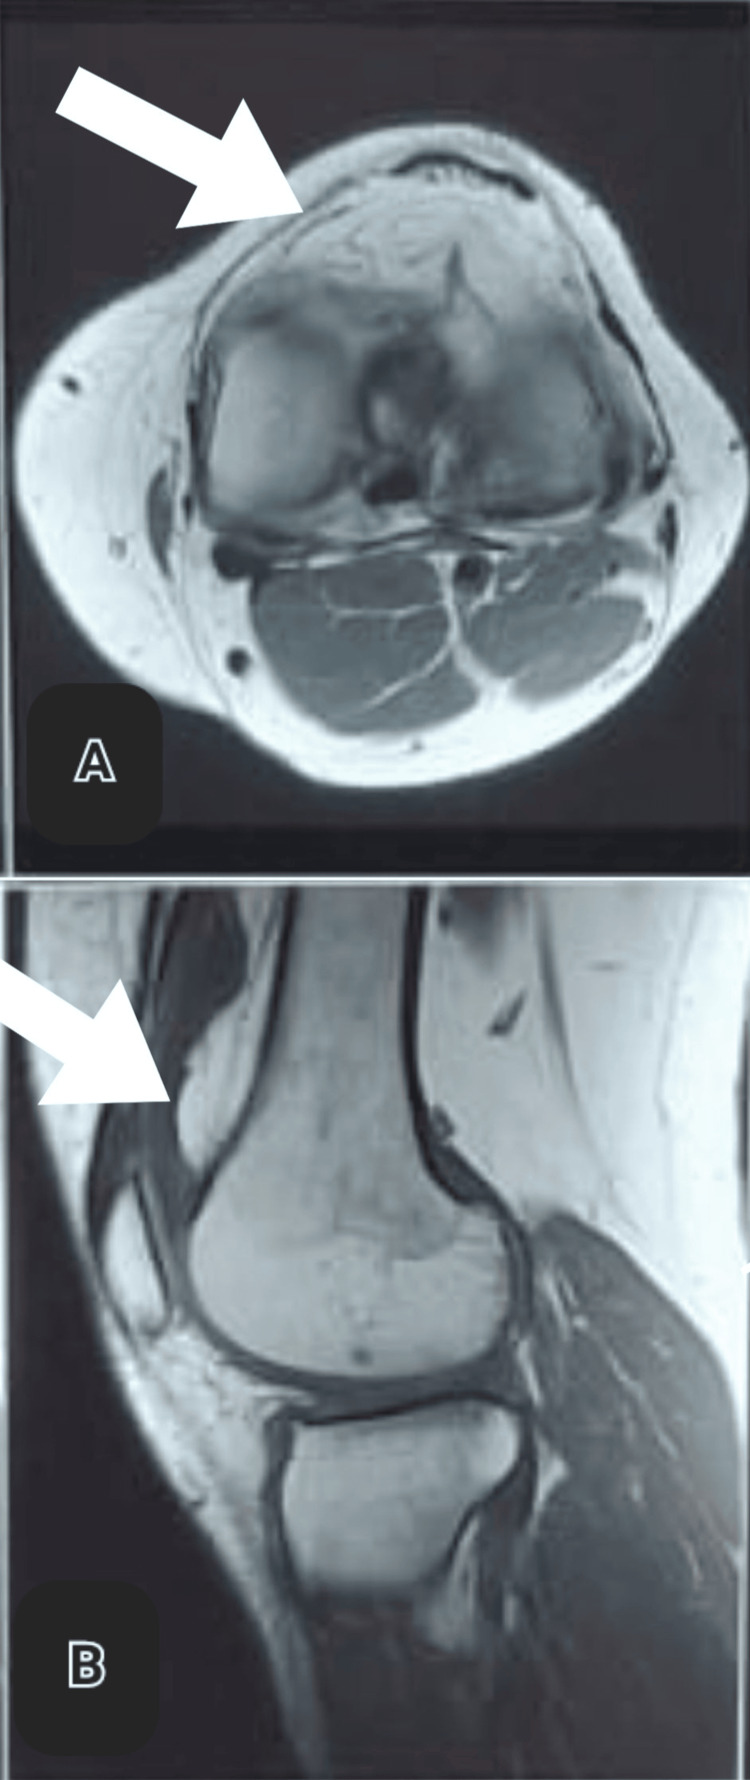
MRI images of the pathology A: axial image, B: sagittal image Arrows indicate the frond-like arborescent synovial mass. MRI: magnetic resonance imaging
